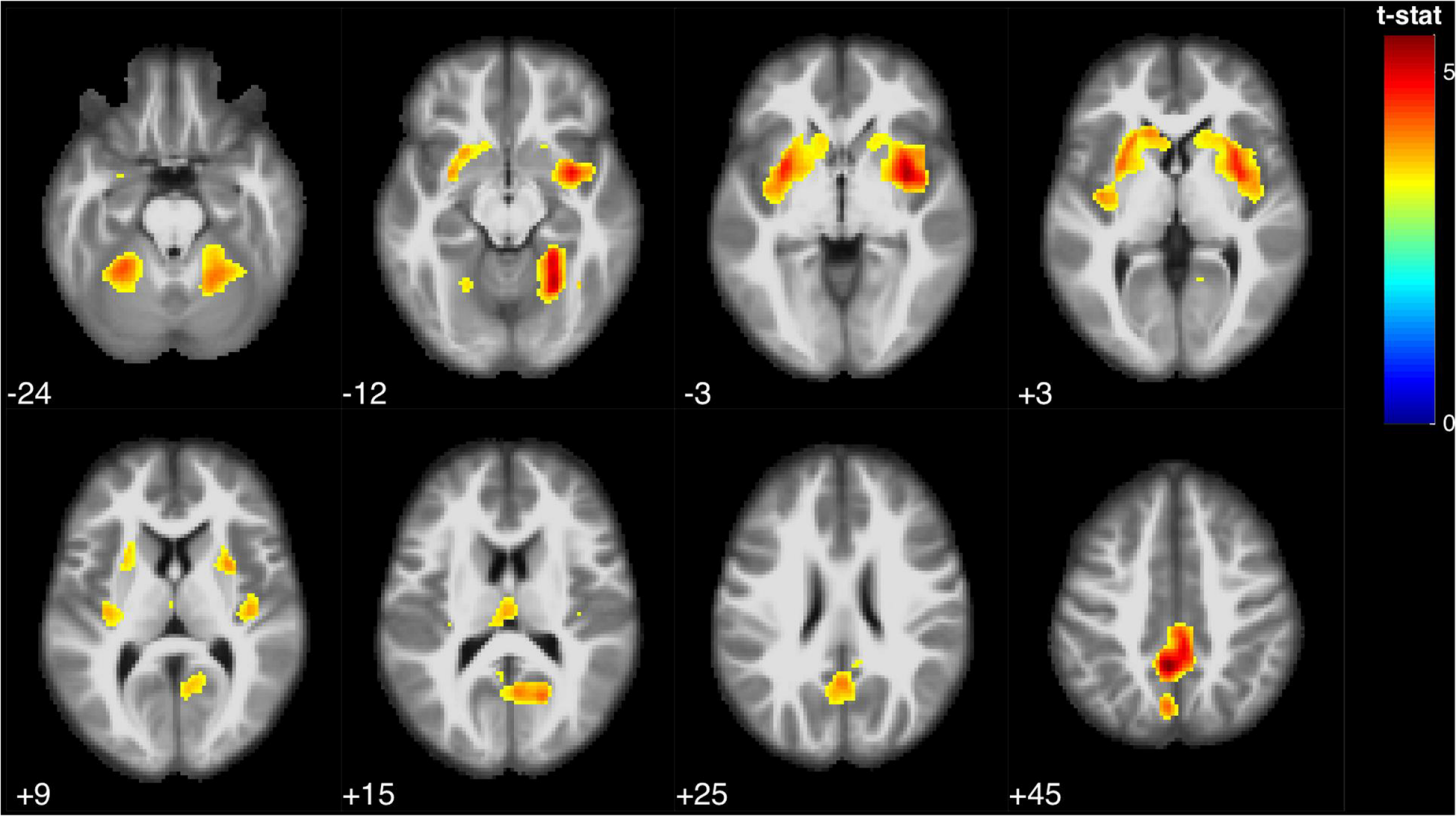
Statistical parametric map showing brain regions positively correlated with PASAT performance (FDR *p* < 0.05, k = 100), rendered on MRI template with neurological orientation
